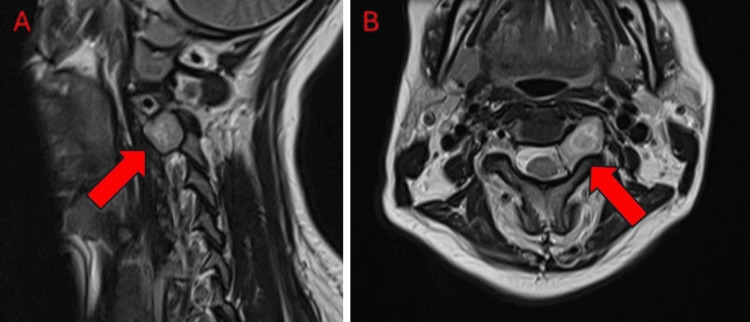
ZAP-X treatment planning MRI images of Patient 1 T2-weighted MRI images of Patient 1, sagittal (A) and axial (B) sections, showing 2.1x1.2x1.6 cm schwannoma. Red arrows indicate cervical schwannoma.
